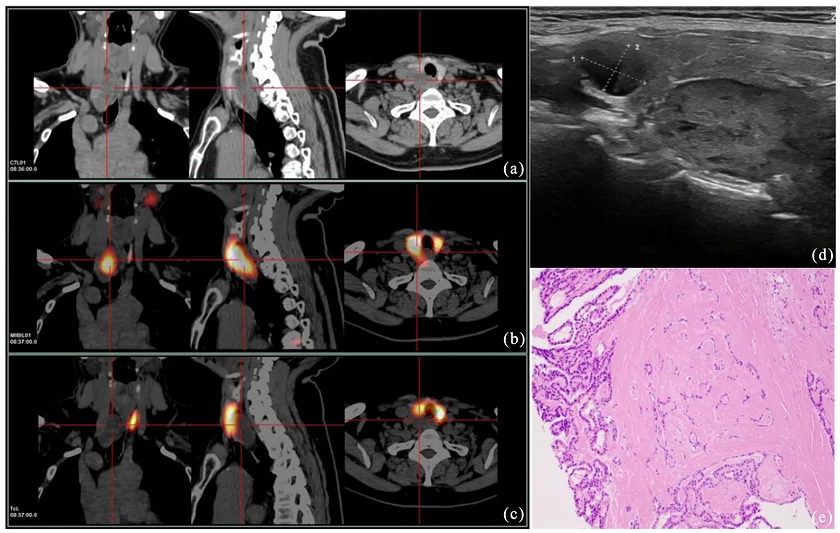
A patient has been in the hospital for nearly a month due to fatigue, dizziness, and poor appetite. Dual‐isotope SPECT/CT imaging: A round‐like soft tissue density lesion is visible on CT (A), with the lesion showing positive on ^99m^Tc‐MIBI images (B) and negative on ^99m^TcO_4_
